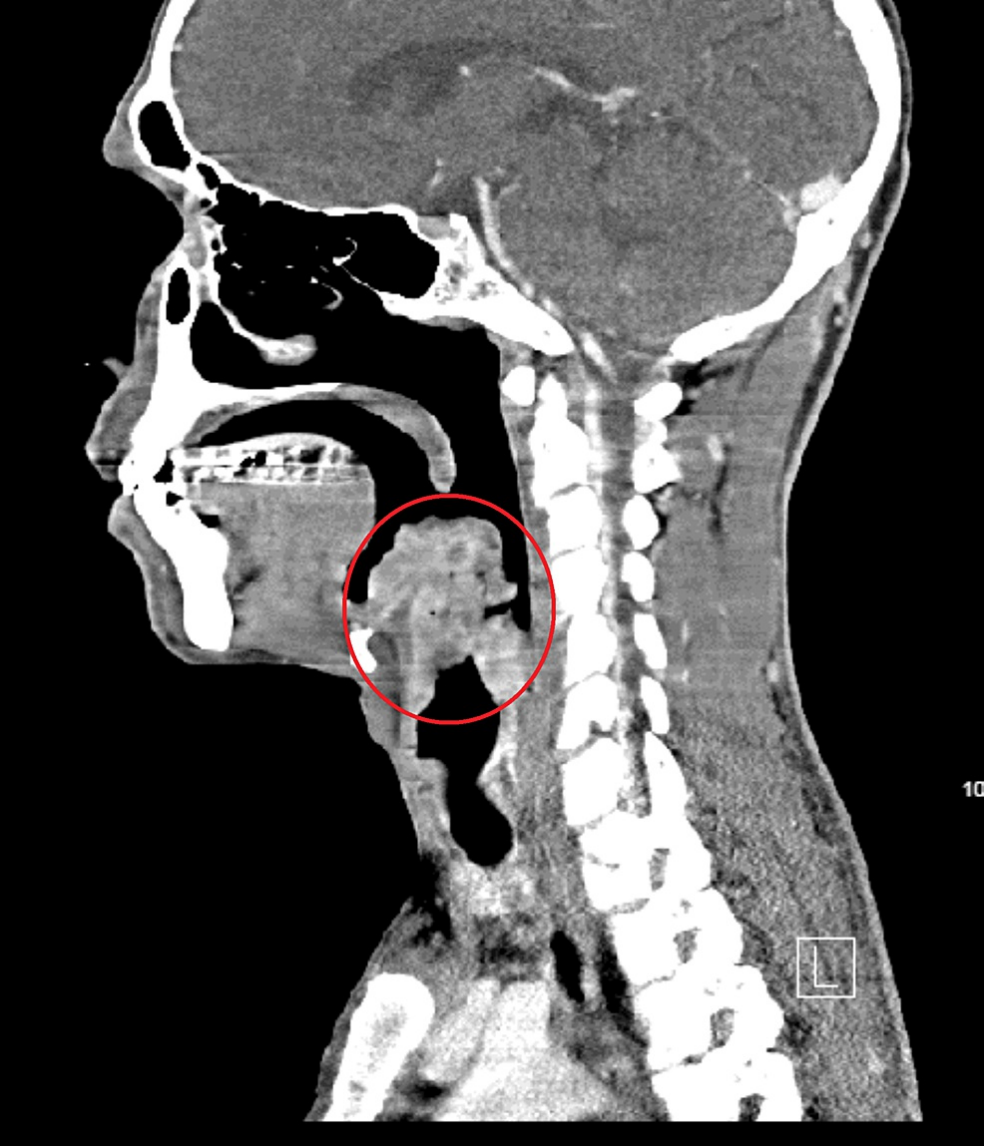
Computed tomography of the soft-tissue neck with contrast sagittal section (lesion in the circle).
